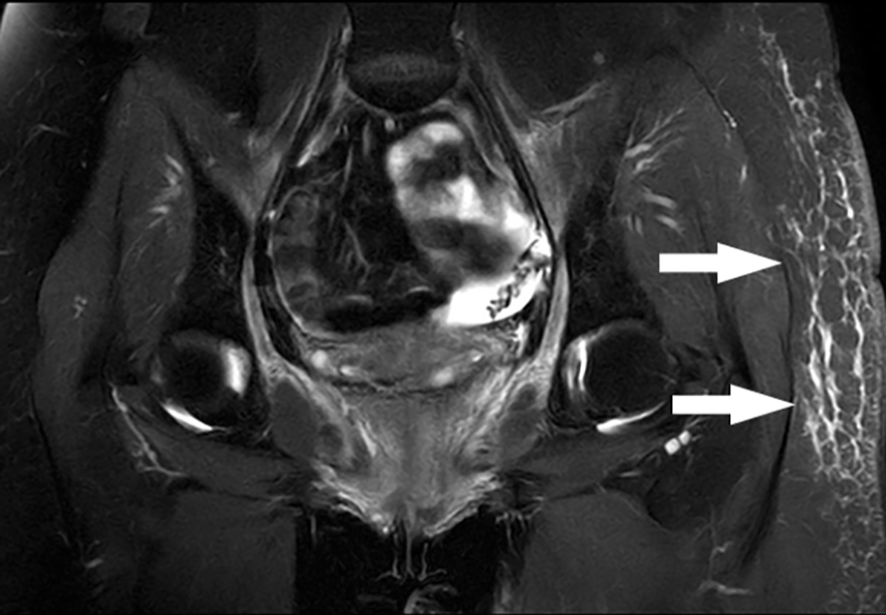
A 34-year-old female with cervical cancer developed left lower limb edema one year following surgery and pelvic radiation therapy. After administration, the pelvic MRI indicated no evidence of cancer recurrence or extrinsic compression, and left thigh subcutaneous tissue edema was observed (white arrow).
